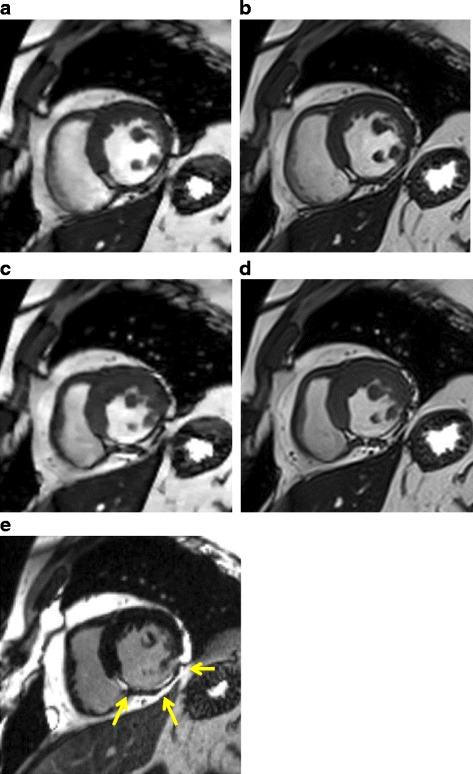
A 69-year-old patient with an inferior wall infarction. a, c Compressed sensing cine CMR images. b, d Standard cine MR images. e Late Gadolinium Enhancement (LGE) CMR images. The images depict the mid short-axis view a and b in the end-diastolic phase and c and d in the end-systolic phase. Both cine images show thinning and akinesia of the inferior myocardium. The LGE CMR shows a transmural infarction in the inferior myocardium (arrows)
